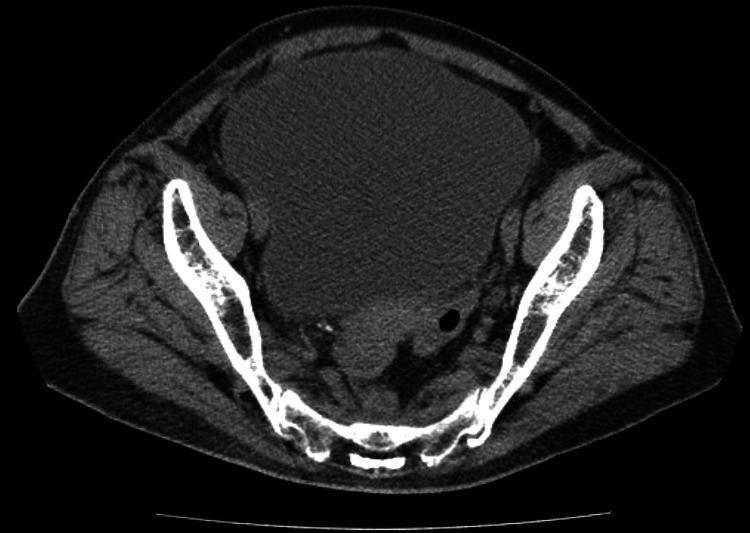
Abdominal CT on admission Abdominal CT revealed severe bladder distention
